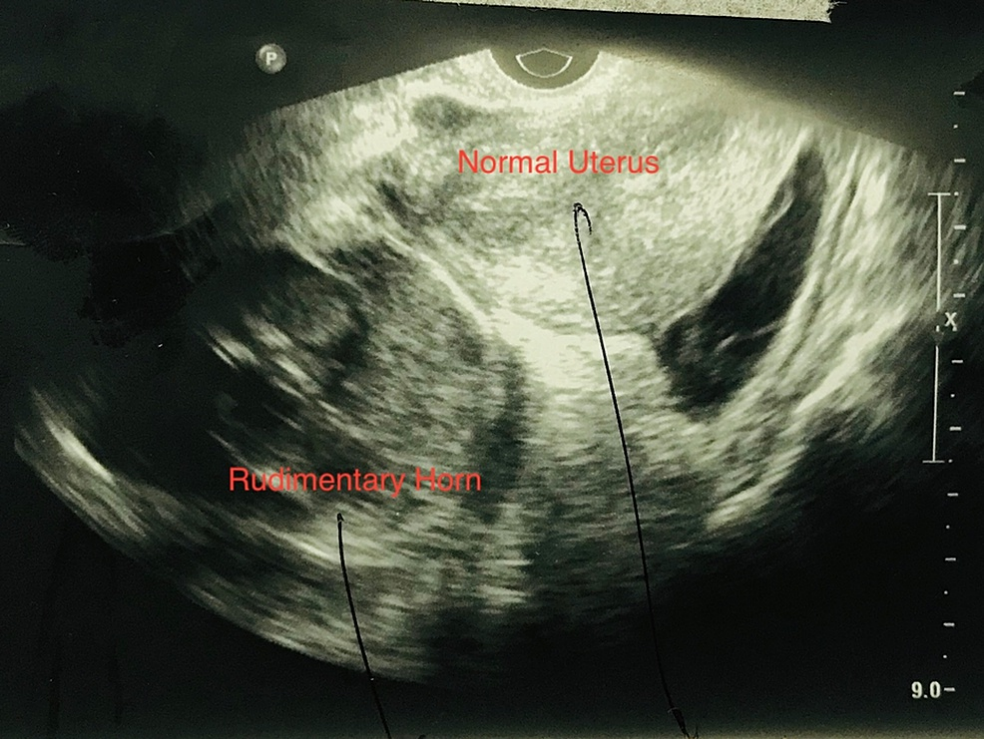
Ultrasound image showing normal uterus with ruptured rudimentary horn with a fetus surrounded by myometrium of the rudimentary horn
